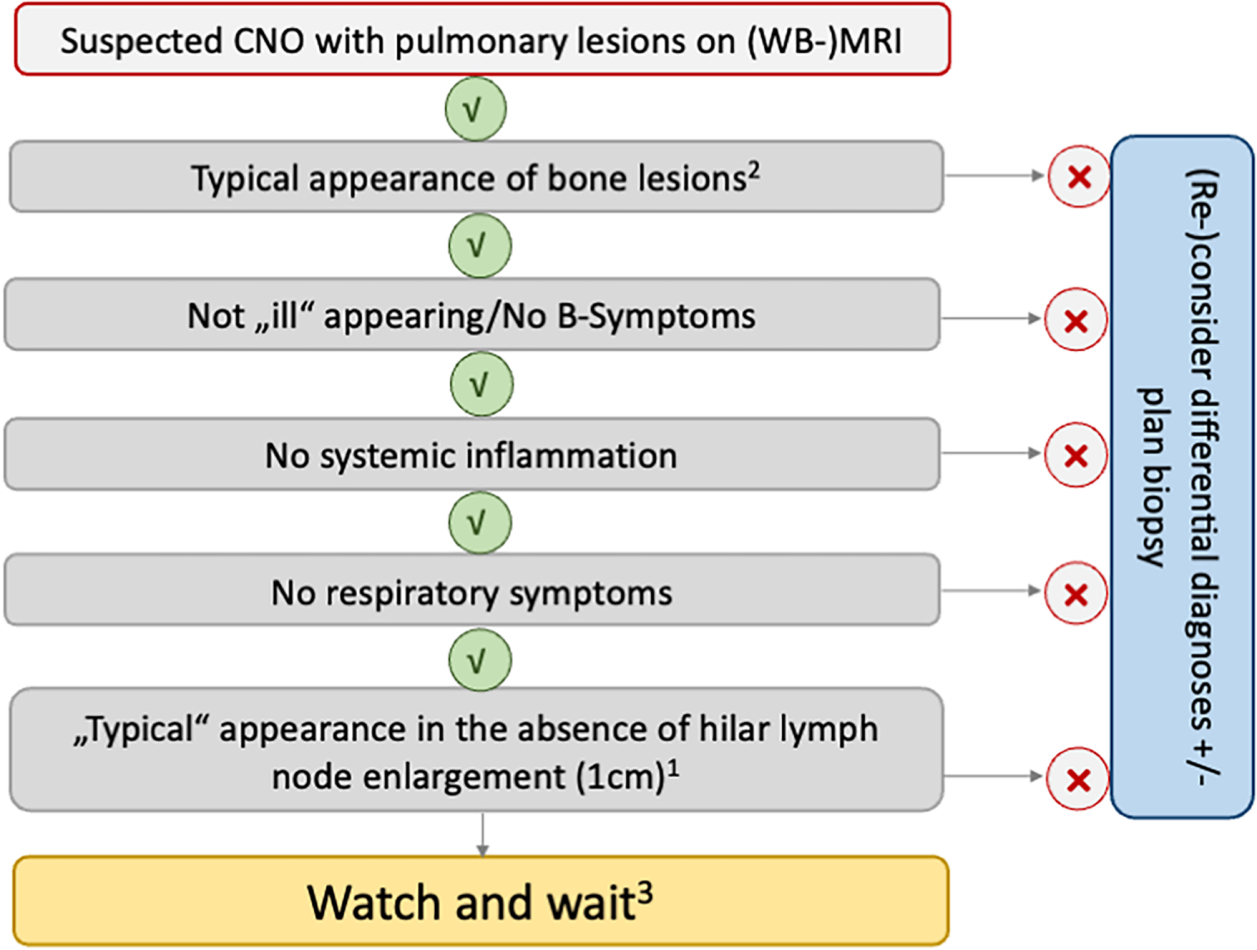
Proposed algorithm for decision‐making in patients with suspected CNO with pulmonary lesions. ^1^Moderately sized (<2 cm) mono‐ or oligofocal lesions, consolidations, nodules ± pleural abutting, hilar lymph nodes not pathologically enlarged (<1 cm). ^2^Polyfocal, symmetric, epi‐/metaphyseal, vertebral, clavicular and/or mandibular involvement, no neurocranial involvement. ^3^Close clinical monitoring (including imaging). CNO, chronic nonbacterial osteomyelitis; WB‐MRI, whole‐body magnetic resonance imaging.
